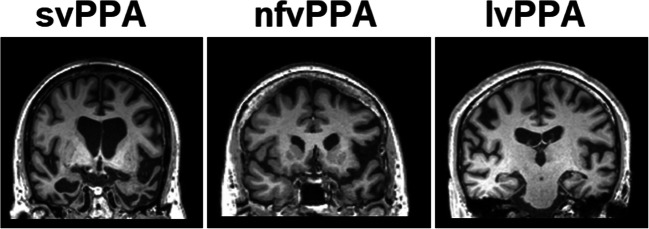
Neuroanatomical profiles of the major syndromes of primary progressive aphasia. Panels present T1-weighted coronal brain MRI sections of patients with typical syndromes of semantic variant primary progressive aphasia (svPPA), nonfluent/agrammatic primary progressive aphasia (nfvPPA), and logopenic variant primary progressive aphasia (lvPPA). Brain images are presented with the left hemisphere on the right. The svPPA scan shows asymmetric (predominantly left-sided) anterior inferior and mesial temporal lobe atrophy; the nfvPPA scan shows asymmetric (predominantly left-sided) inferior frontal, insular, and anterior-superior temporal gyrus atrophy, while the lvPPA scan shows asymmetric (predominantly left-sided) temporo-parietal junction atrophy
